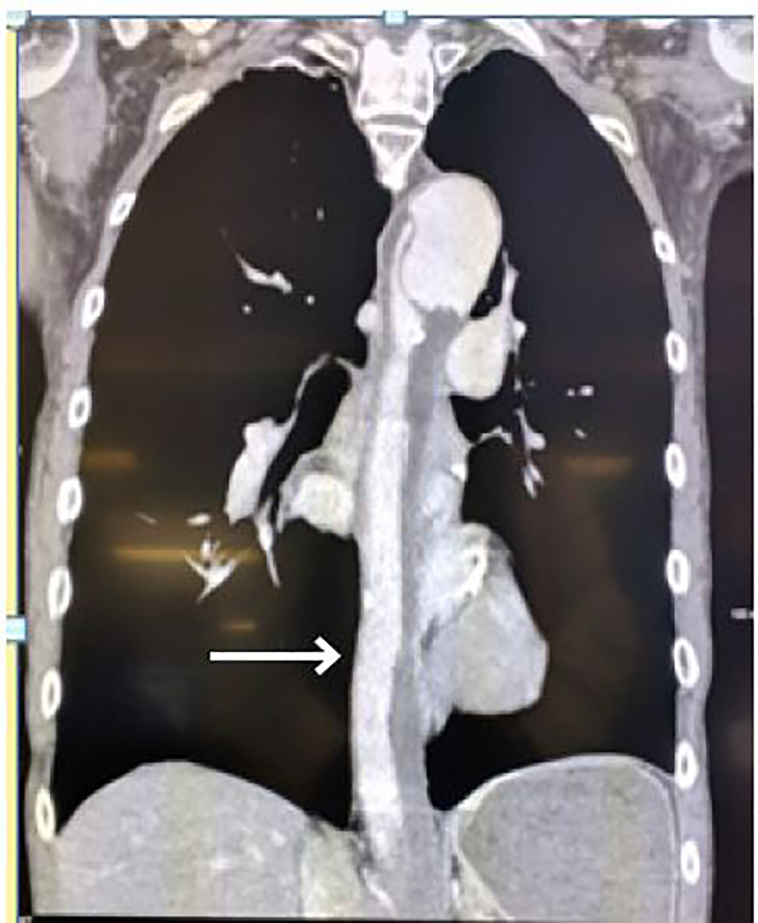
Computed tomography of the chest in coronal view demonstrating a Stanford Type B aortic dissection (arrow).
